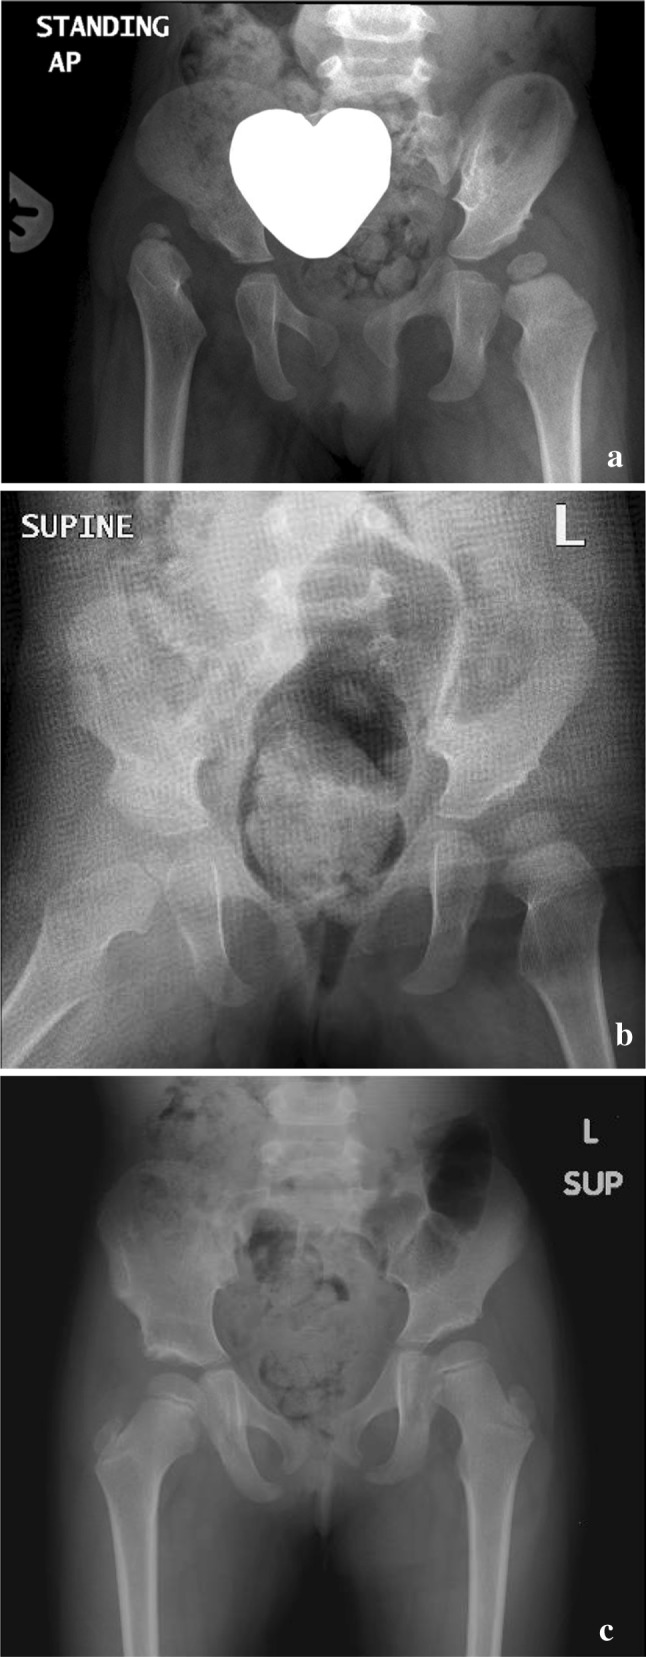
a A 2-year-old girl with right hip dislocation and dysplastic left hip. b Ten weeks after right hip open reduction and acetabuloplasty. c Follow-up radiograph postoperatively at 2 years, showing maintained right hip reduction and spontaneous improvement of left hip dysplasia
